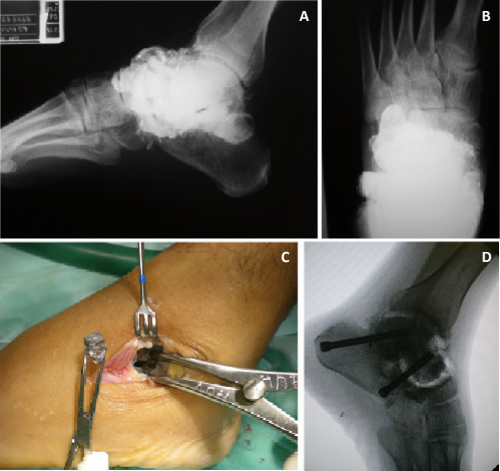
Case 2: A. AP radiograph. Commitment severe subtalar and Chopart joint by gun fire. B. AP radiograph. Commitment severe subtalar and Chopart joint by gun fire. C. subtalar approach. Removing Projectiles and intraarticular fragments lead. D. Lateral Ankle radiograph. Subtalar arthrodesis with compression screws. Liberation Talo-Navicular joint and autografts
